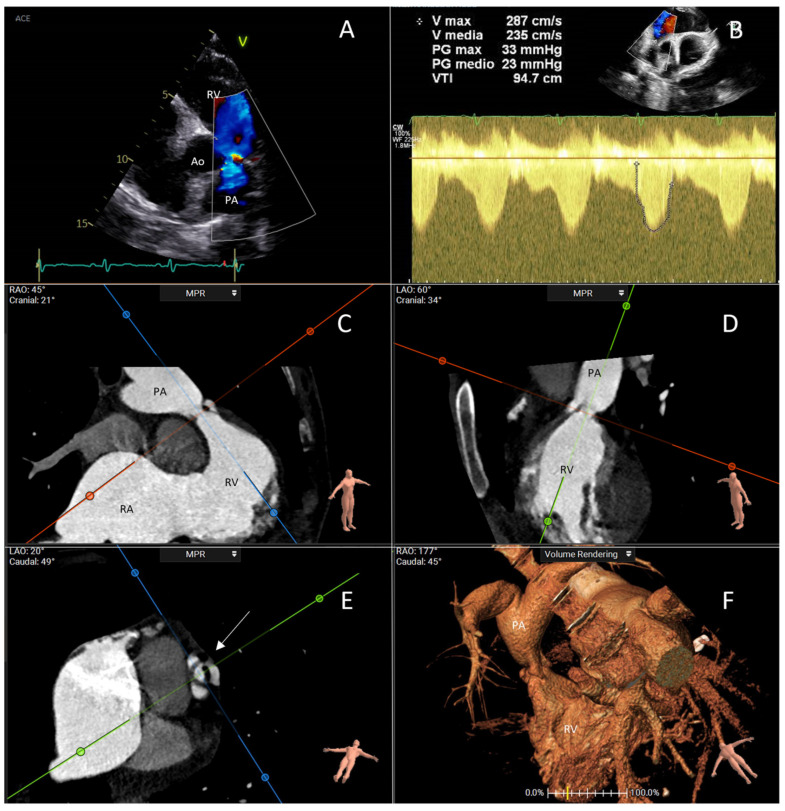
This figure represents a case evaluated at Fondazione Policlinico Universitario Campus Bio-Medico, Rome. It is about a 51-year-old woman who had severe pulmonary valve stenosis and torrential tricuspid regurgitation in the setting of carcinoid heart disease. (A) The transthoracic echocardiogram with color Doppler shows turbulence in blood flow across the stenotic pulmonary valve. (B) Continuous-wave Doppler examination shows a typical curve of severe pulmonary stenosis with increased gradient across the valve; this was a case of low-flow–low-gradient pulmonary stenosis because of the coexistence of torrential tricuspid regurgitation. (C–E) CT scan examination with multiplanar reconstruction in systole reveals the morphology of the pulmonary valve (arrow), showing thickened and hypomobile cusps. (F) Three-dimensional volume rendering showing the narrowed pulmonary valve and the post-stenotic dilation of the pulmonary artery. Ao: aorta; PA: pulmonary artery; RA: right atrium; RV: right ventricle.
